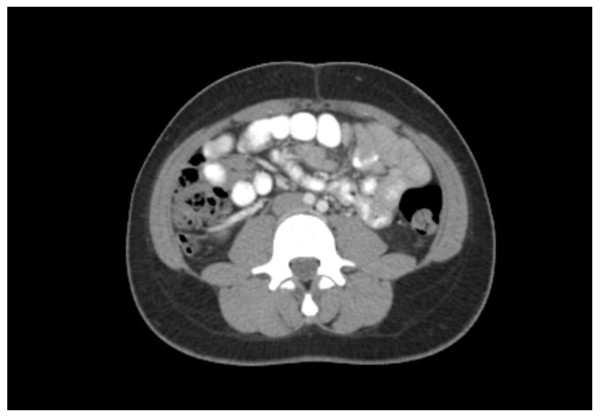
Axial computed tomography images depicting the appendiceal duplication.
